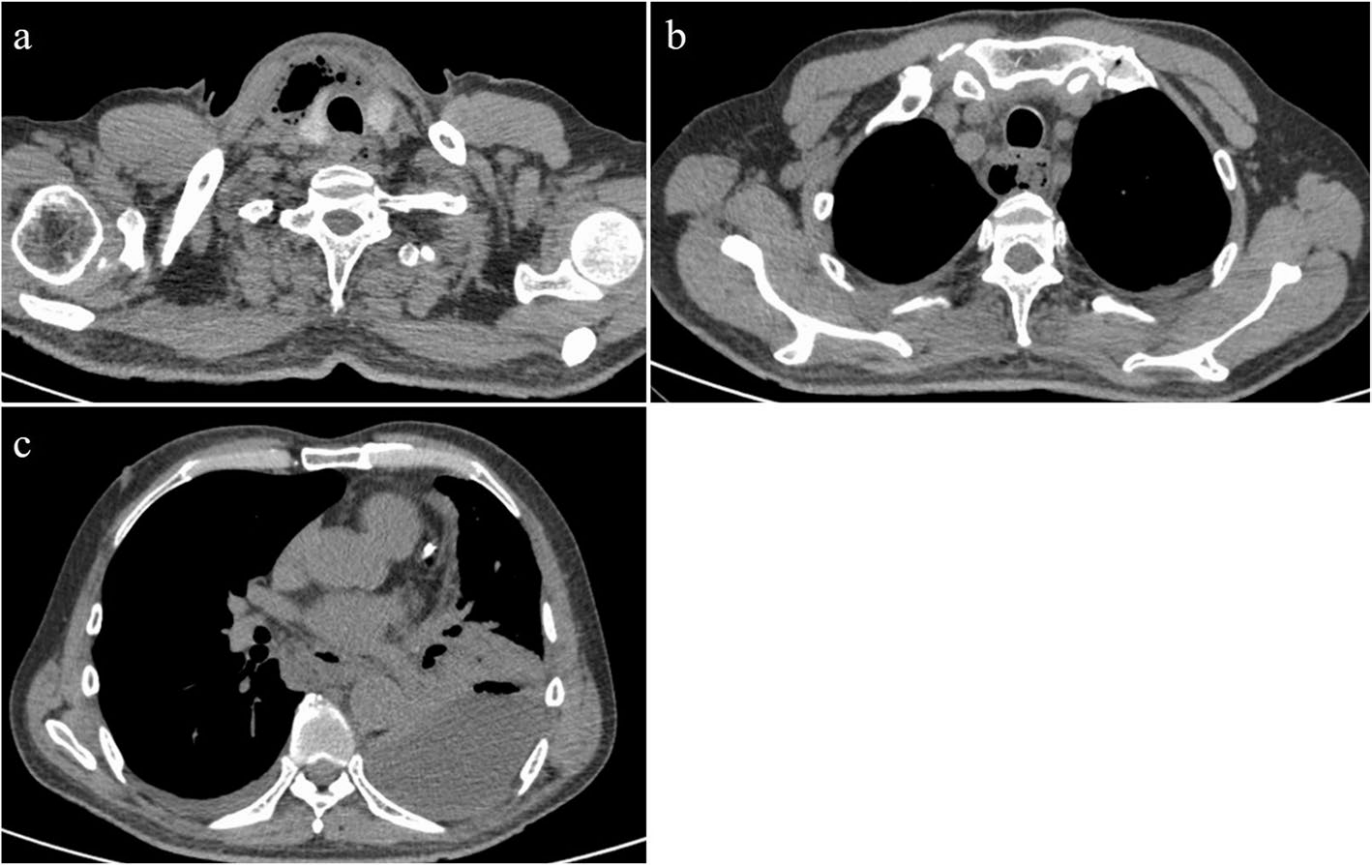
Chest computed tomography (CT) image at 3 days after admission showing gas bubbles and a pool of pus in the cervix (a), gas bubbles in the superior mediastinum (b), and left thoracic effusion (c)
